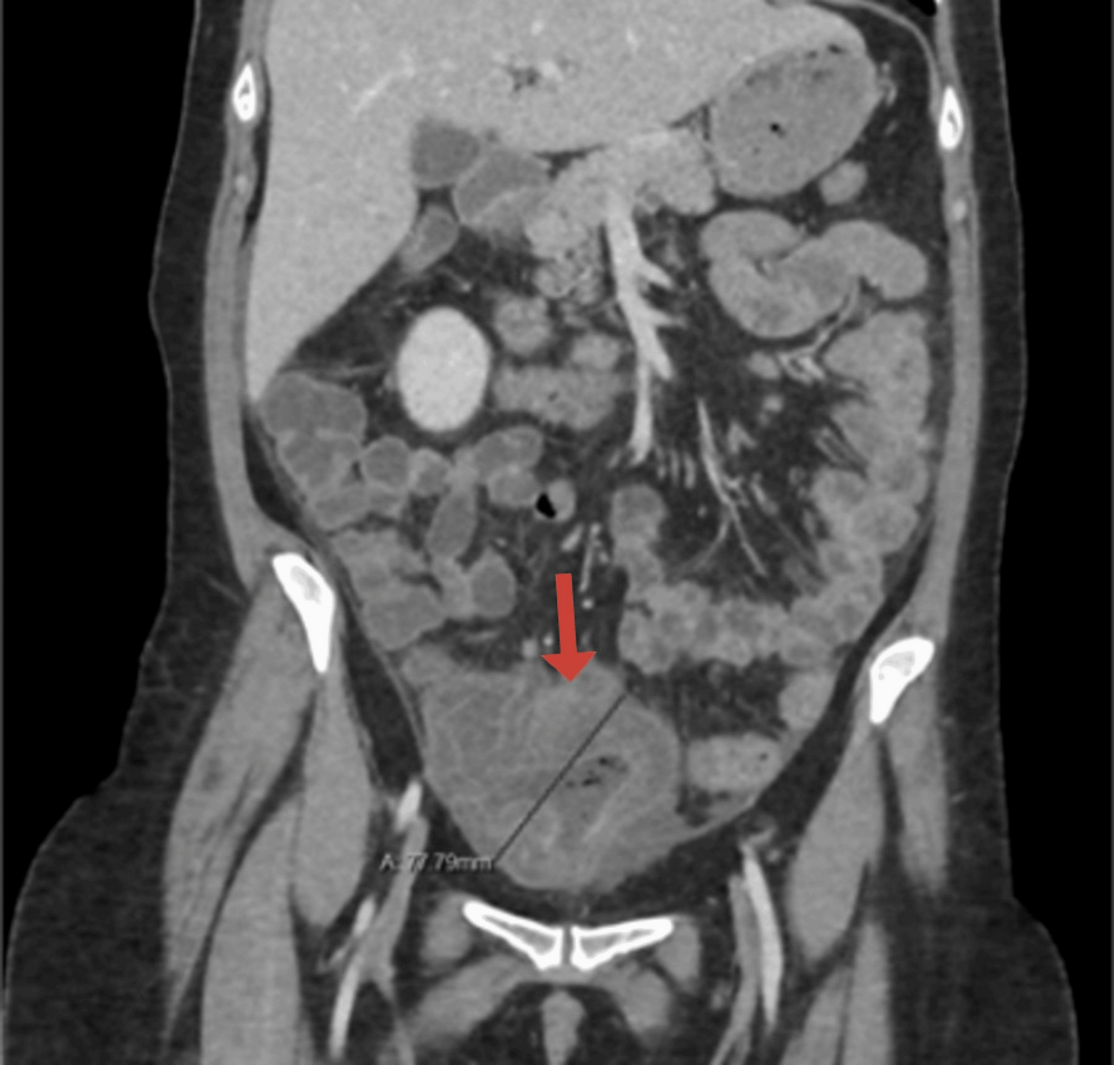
Contrast-enhanced CT of the abdomen (coronal view) Severe thickening and enhancement of the cecal wall with submucosal edema (red arrow), measuring up to 2.2 cm in thickness.
